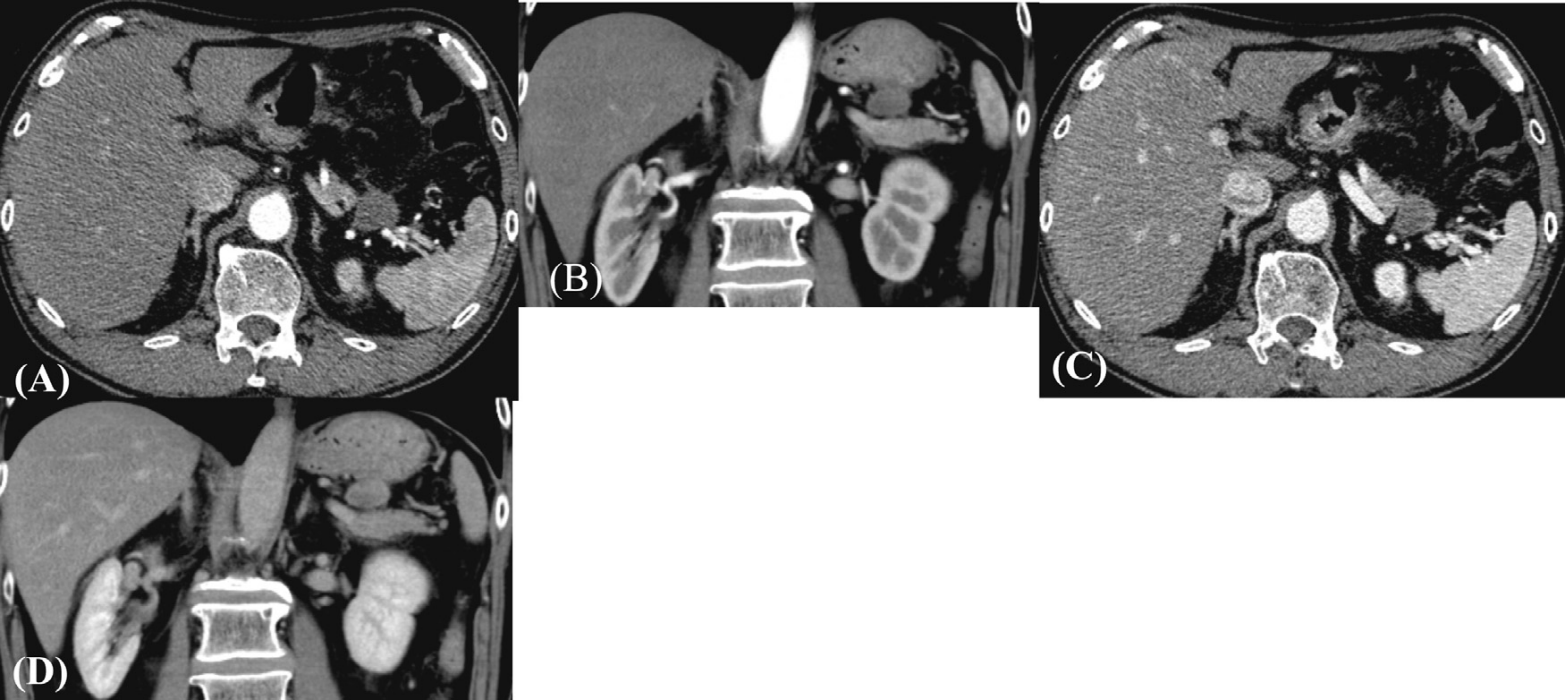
Contrast-enhanced CT findings 6 months after initial CT. Early phase axial section (A) and coronal section (B). Late phase axial section (C) and coronal section (D). The mass suspected to be of retroperitoneum origin and was growing slightly. It gradually enhanced from early phase to late phase with mild enhancement. The enhanced pattern was heterogeneous; namely, the lower half of the mass had weaker enhancement.
